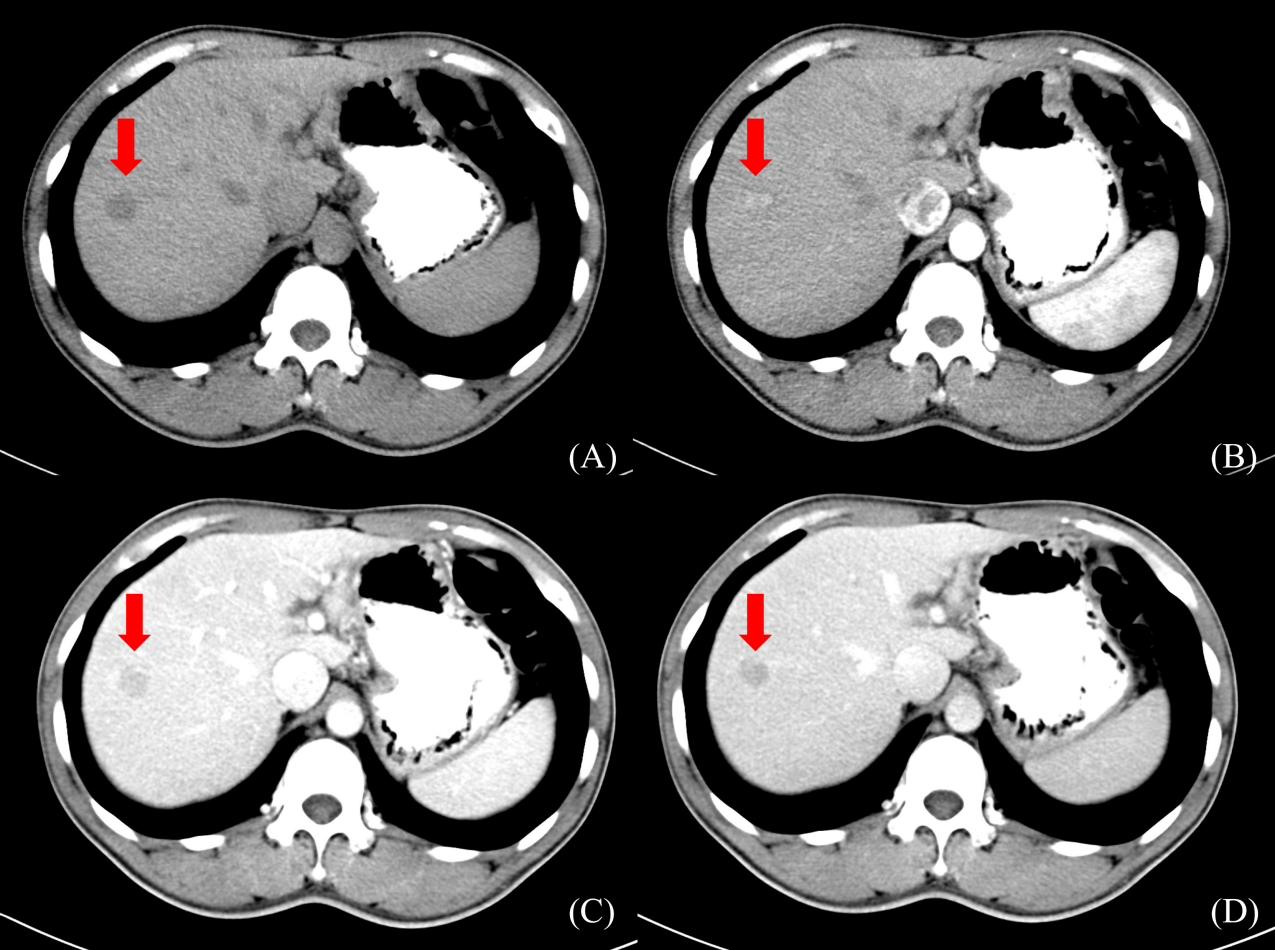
HCC CT border imaging. (A) CT scan without contrast (B) CT contrast scan in arterial phase (C) CT contrast scan in portal venous phase (D) CT contrast scan in delayed phase.
